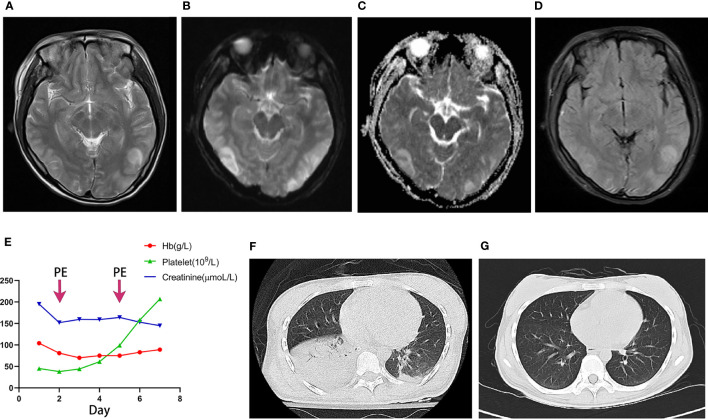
(A–D) MRI of the patient’s brain, multiple lesions in the bilateral frontoparieto-occipitotemporal lobe and left basal ganglia region: (A) T2 weighted image; (B) Diffusion Weighted Imaging (DWI); (C) Apparent Diffusion Coefficient (ADC); (D) Fluidattenuated Inversion Recovery (FLAIR). (E) Changes in hemoglobin, platelets and creatinine from day 1 to day 7, with the arrows referring to the time of plasma exchange therapy. (F) HRCT of the patient's chest at the time of admission. (G) Chest HRCT on day 7.
